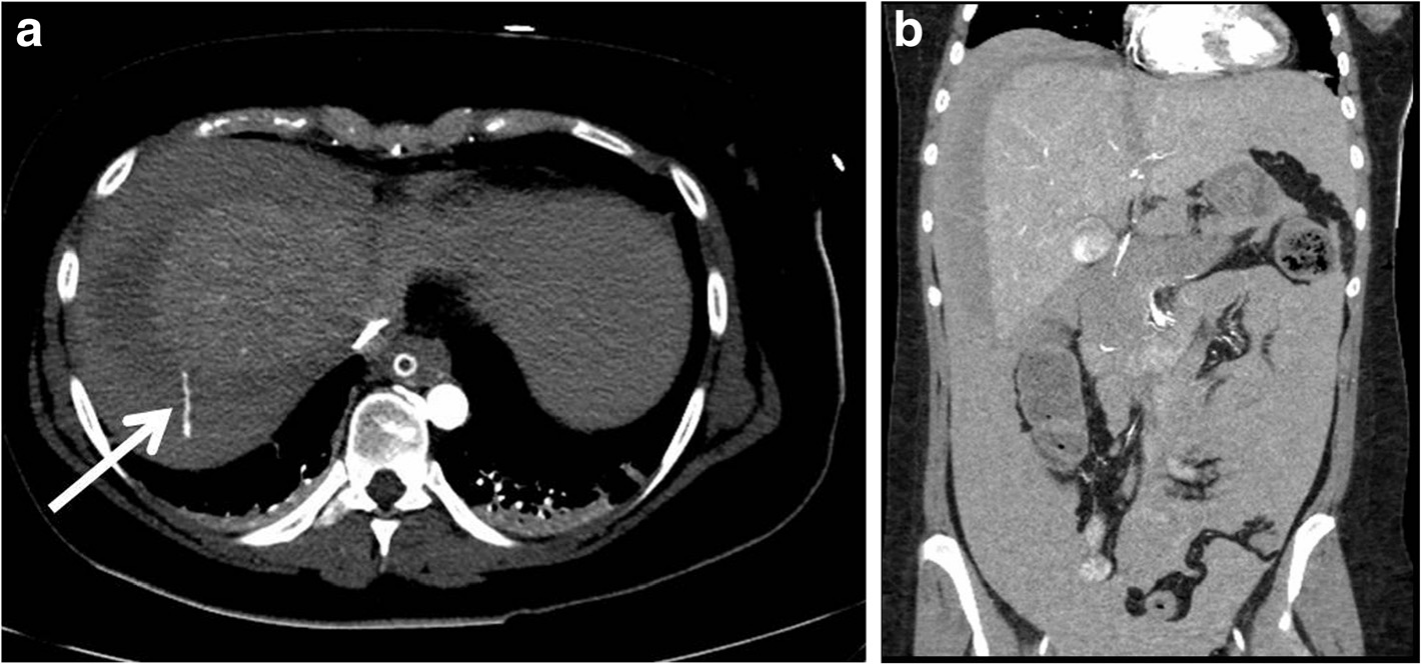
Axial (a) and coronal (b) computed tomographic images of the abdomen revealing active contrast extravasation from the liver (*arrow*) and a massive hemoperitoneum
